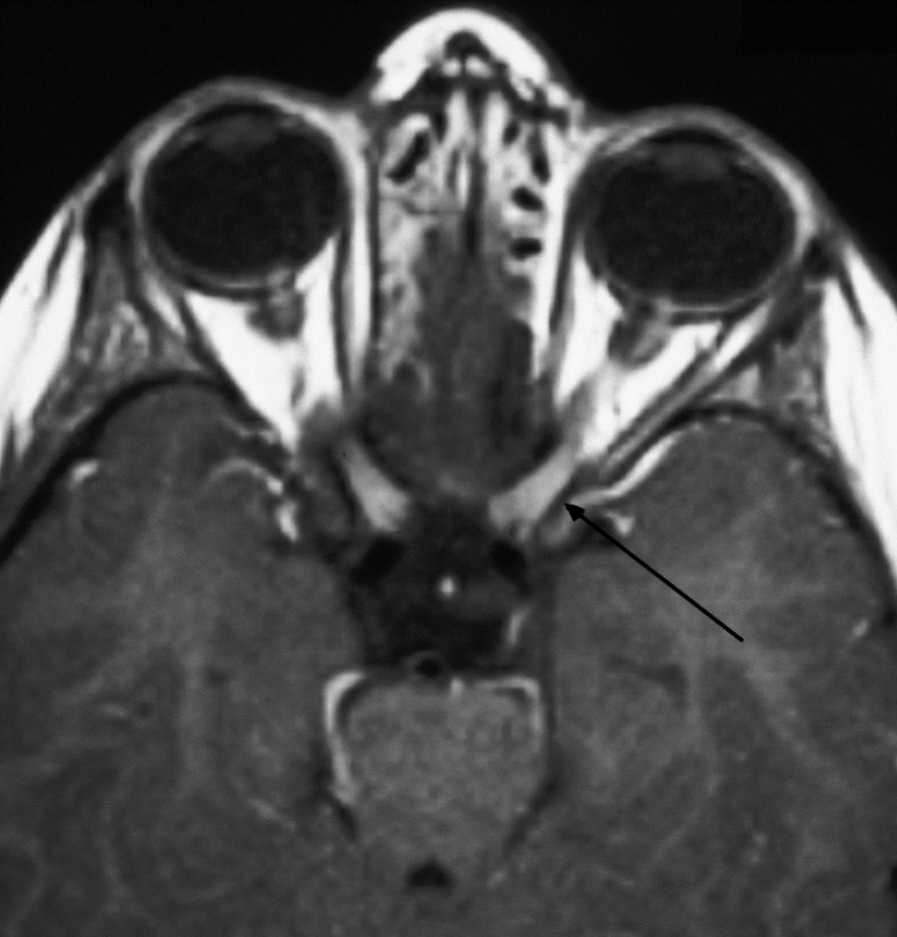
MRI brain showing hyperintensity in the right optic nerve head
